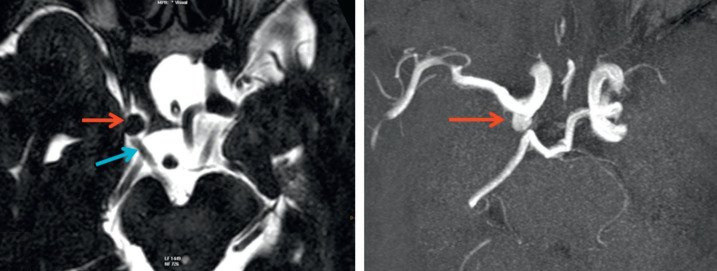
MRI axial T2 (a) and TOF (b). Posterior communicating artery aneurysm compressing the IIIrd oculomotor nerve (from Vignal-Clermont C, Tilikete C, Milea D. Neuro-ophtalmologie. 2e edition, 2016, Elsevier)
